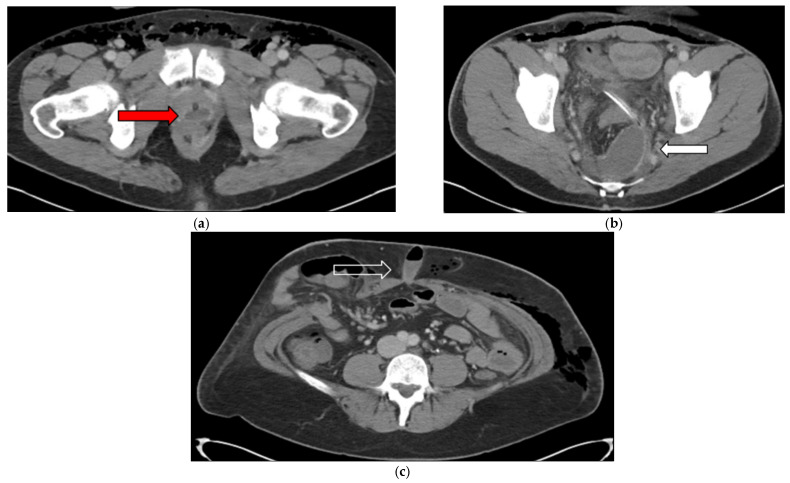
(a–c) Postoperative contrast-enhanced computed tomography images demonstrating multiple fluid collections. (a) Fluid collection posterior to the prostate, measuring 2.9 cm (red arrow). (b) Fluid collection in the left presacral region, measuring 6.2 cm (white arrow). An operative closed-suction drain is also visible. (c) Fluid and gas collection at the umbilicus, measuring 4.2 cm (white outlined arrow).
